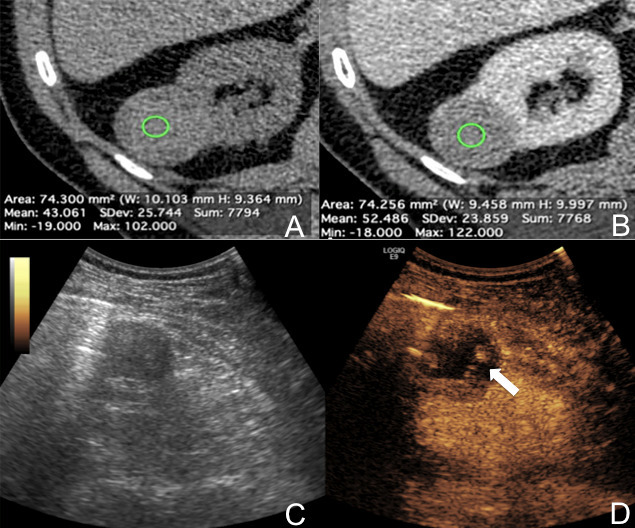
CEUS-induced reclassification to Bosniak 3 cyst of a right-sided IL found on CT. The round renal mass with partially ill-defined margins showed soft-tissue attenuation on basal unenhanced scan (values in the circular ROI in A). However, no significant contrast-enhancement was found, as shown by an increase in attenuation values < 20 HUs (values in the ROI in B). B-mode ultrasound (C) found a largely anechoic lesion, with multiple, small solid nodules better appreciated as enhancing areas after microbubble contrast administration (arrow in D). The lesion was proven to be clear cell renal carcinoma after surgical resection. CEUS, contrast-enhnaced ultrasound; HU, Hounsfield unit; IL, indeterminate lesion; ROI, region of interest.
